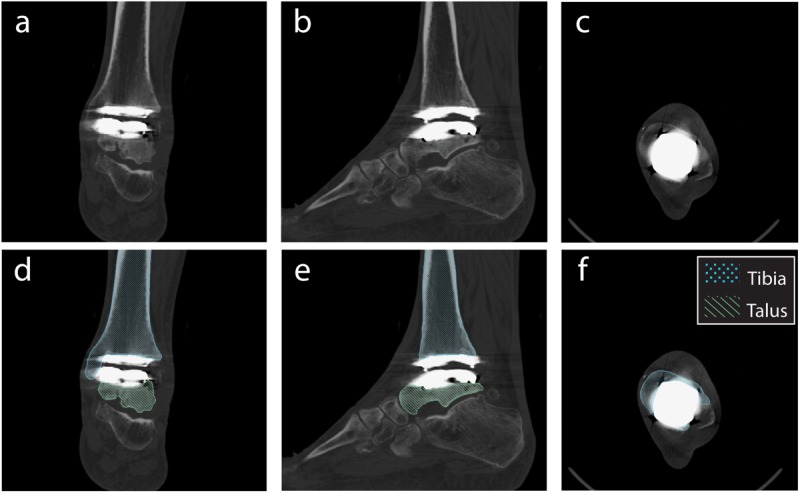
Planar CT image slices using the metal artifact reduction algorithm (Siemens iMAR^®^). Planar views demonstrated in: (a,d) Coronal, (b,e) Sagittal, and (c,f) Transverse planes. Segmentation shown for the tibia and talus (d–f).
